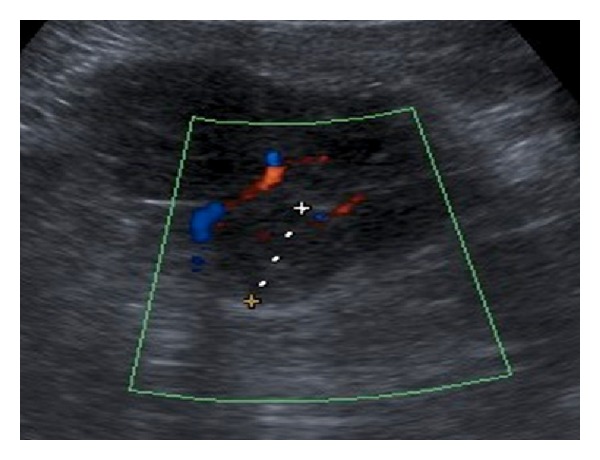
Renal ultrasound demonstrates an exophytic hypoechoic solid mass arising from the lower pole of the kidney consistent with a renal cell carcinoma.
